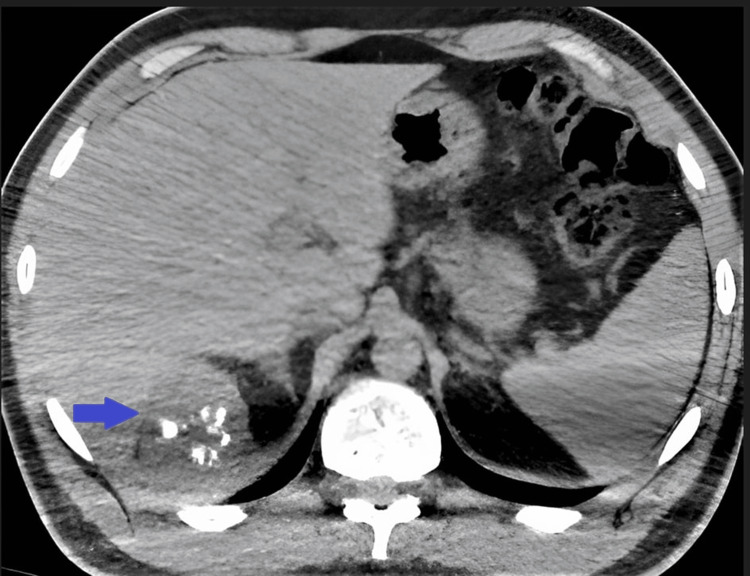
Unenhanced computed tomography image in the axial plane of a centrally calcified retroperitoneal mass localized between the diaphragm and liver.
